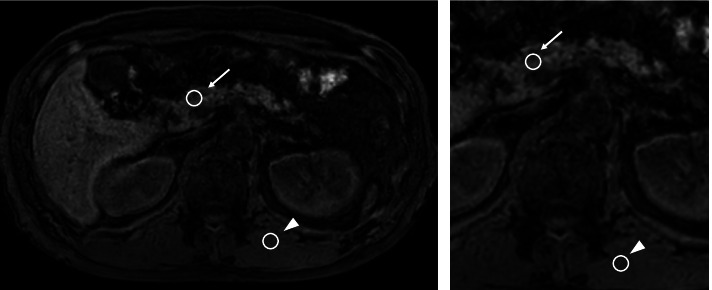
The pancreas-to-muscle signal intensity ratio on fat-suppressed axial T_1_-weighted MRI was calculated by [signal intensity of the pancreatic parenchyma] (arrow)/[signal intensity of the paraspinal muscle] (arrowhead)
